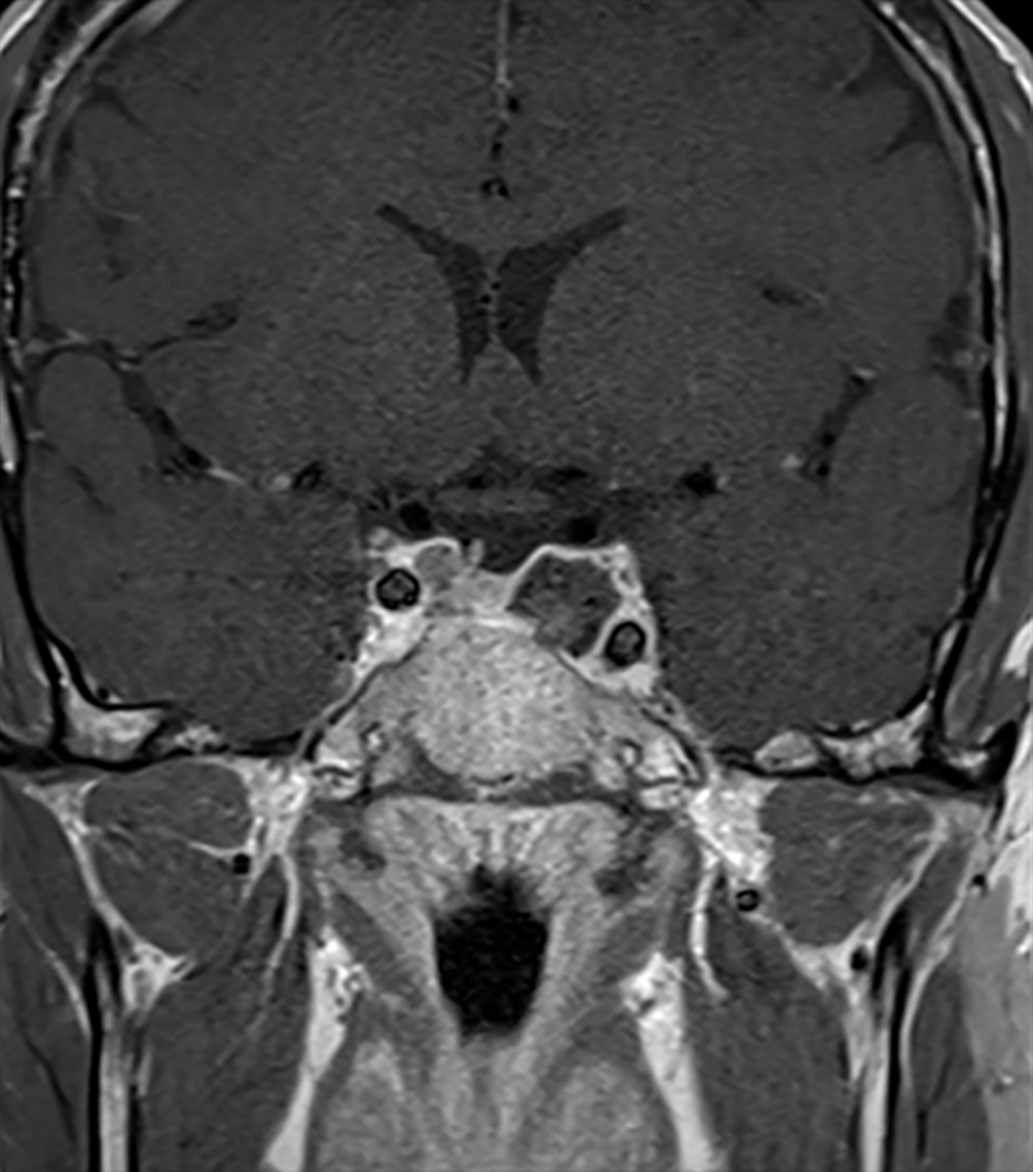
Post-operative MRI. Sagittal MR Imaging T1 with Gadolinium showed a total resection of the pituitary adenoma.
